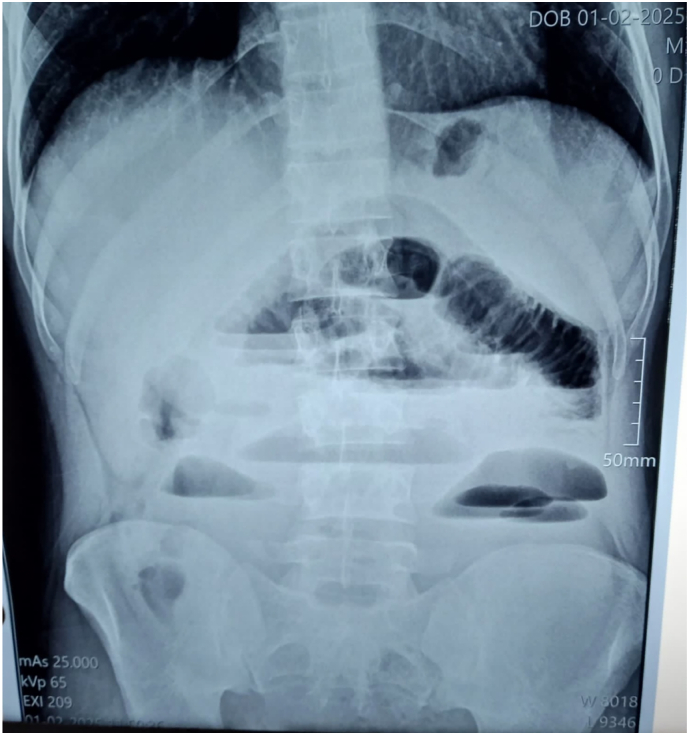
The abdominal X-ray image in the standing position shows the presence of gas-fluid levels with a complete absence of gas in the pelvis. There is no gas crescent under the diaphragm.
